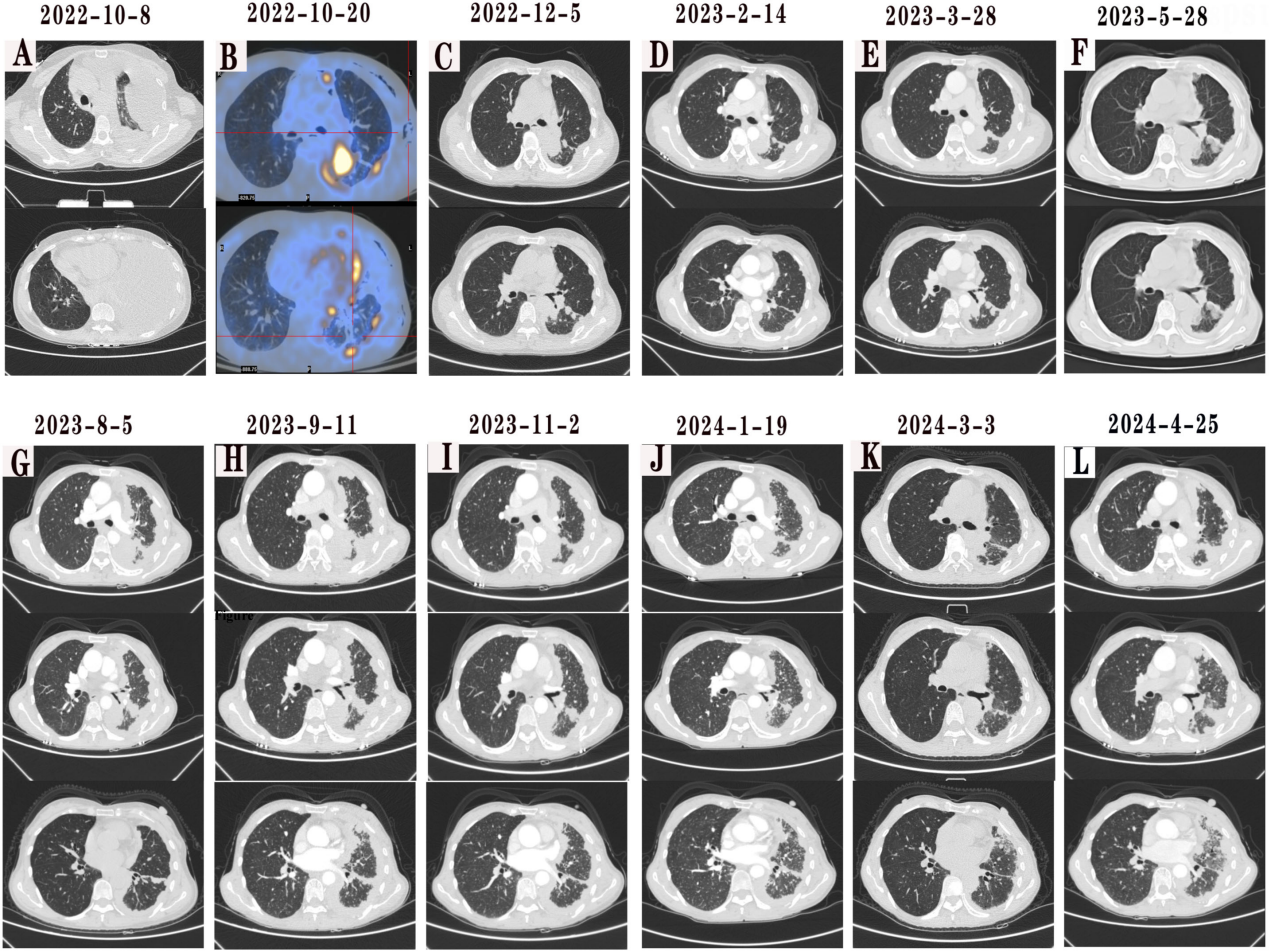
(A) On admission (October 8, 2022), CT scan showed left pneumonia with massive effusion of the left lung and left lung atelectasis. (B) On October 20, 2022, 18F-FDG PET-CT scan were performed after thoracoscopic surgery and showed soft tissue density mass near the left upper lung portal. (C–F) After starting first-line treatment with gefitinib, chest CT scans on February 14, 2023, March 28, 2023, and May 28, 2023, showed that tumor size was similar to that on December 5, 2022. (G) On August 5, 2023, chest CT scan showed left hilar carcinoma with left pleural tumor metastasis and left pleural effusion progression. (H–J) From September 2023, the patient was switched to osimertinib as second-line drug, on November 2, 2023 chest CT scan indicated slow progression of the chest lesion, while January 19, 2024 chest CT scan showed that the metastatic pulmonary nodules in the right lung were found to increase significantly. (K) On February 2, 2024, we replaced the patient with dacomitinib for treatment, on March 3, 2024 chest CT scan showed that the metastatic tumors in the left pleura and the metastatic tumors in the right lung were found to shrink significantly. (L) However, on April 25, 2024, a follow-up chest enhanced CT scan showed that the lesion had progressed again.
